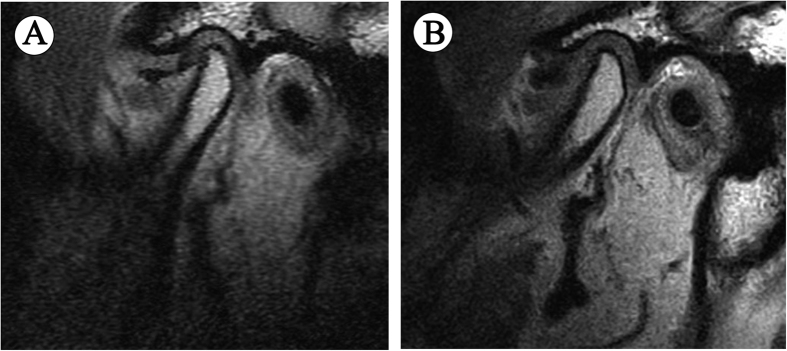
MRI scans of a 55-year-old male ADDWoR patient with the interval of 11 months, showing significant deterioration of disc status. (A) First visit, (B) Follow-up visit.
